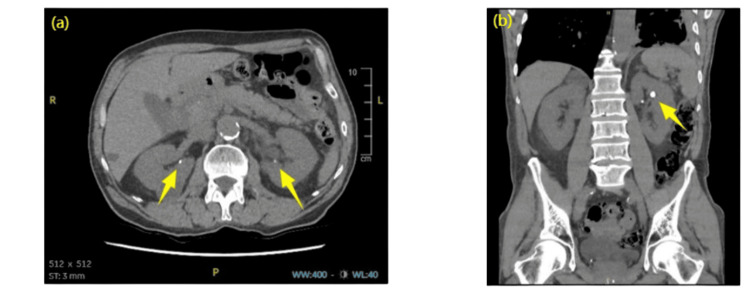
Computed tomography (CT) scan images showing (a) axial section with small non-obstructing calculi visible in the bilateral renal pelvis (yellow arrowhead) and (b) coronal section showing more prominent two small left renal pelvic calculi (yellow arrowhead).
